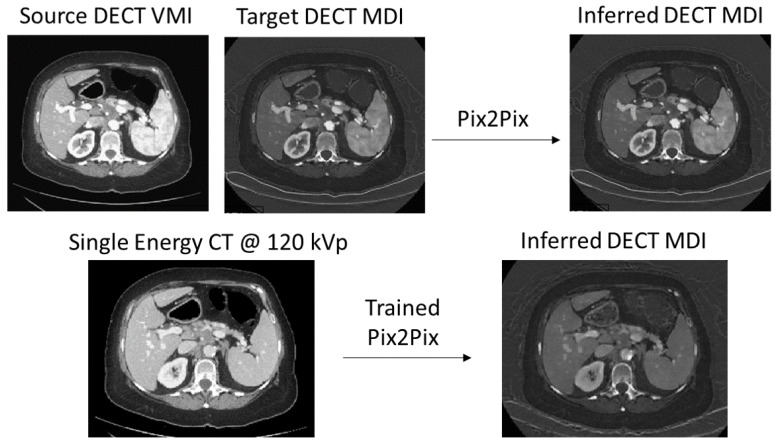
The Pix2Pix system was trained to map dual-energy CT virtual monochromatic images (DECT VMI) reconstructed at 70 keV to DECT material density iodine (MDI) images. Then, the trained system is used to convert single energy CT (SECT) scans acquired at 120 kVp to the synth-DECT MDI image types. Four liver segmentation frameworks were trained and tested with synth-DECT MDI and SECT scans.
